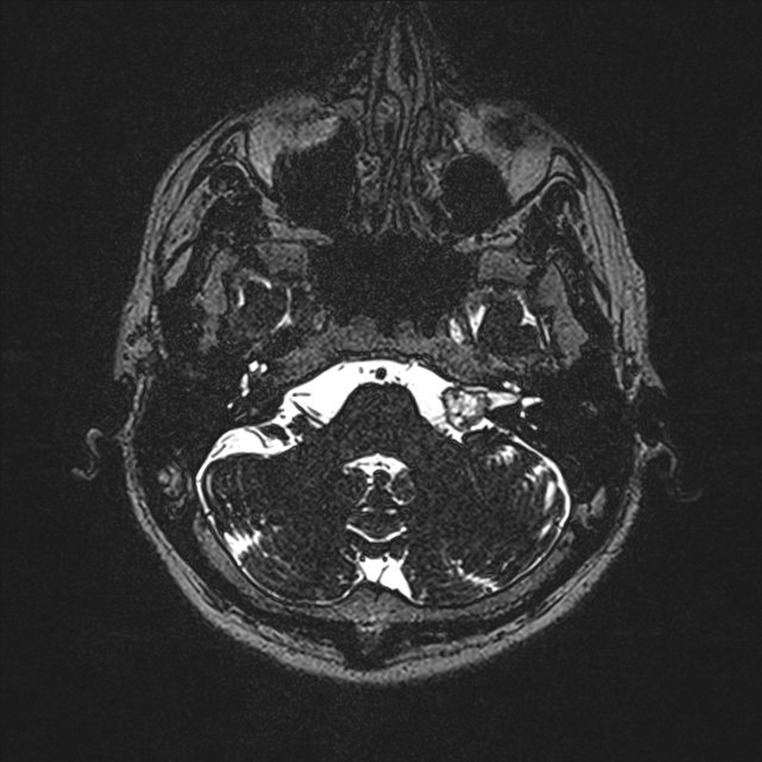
Magnetic resonance image showing lesion in the left pontocerebellar angle.
